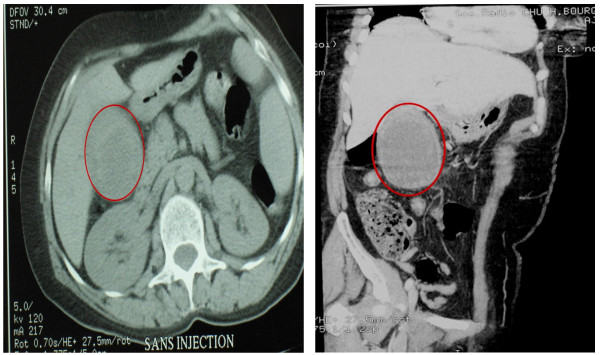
Abdominal computed tomography revealed a solid mass within the gallbladder. 1a and 1b: Abdominal computed tomography revealed a solid mass within the gallbladder, and absence of liver nodules.
